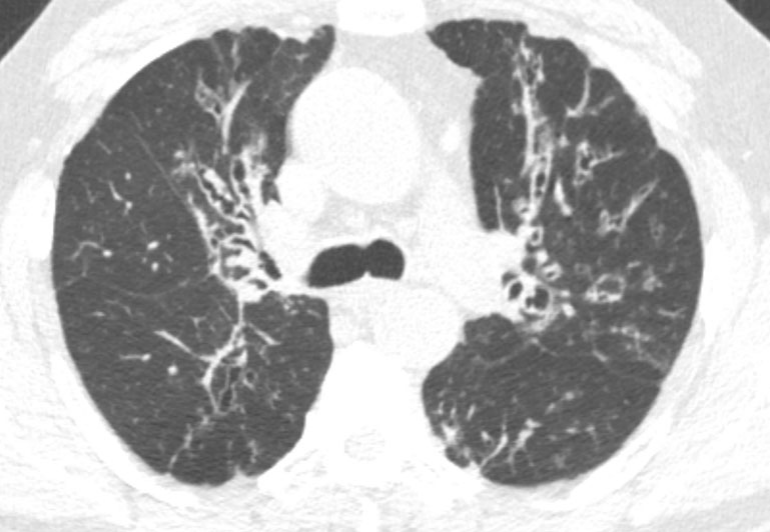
Bronchiectasis due to allergic bronchopulmonary aspergillosis (ABPA). Axial CT image demonstrates the multifocal areas of central bronchiectasis with upper lobe predominance with adjacent areas of atelectasis, ground-glass opacity, and centrilobular nodules
